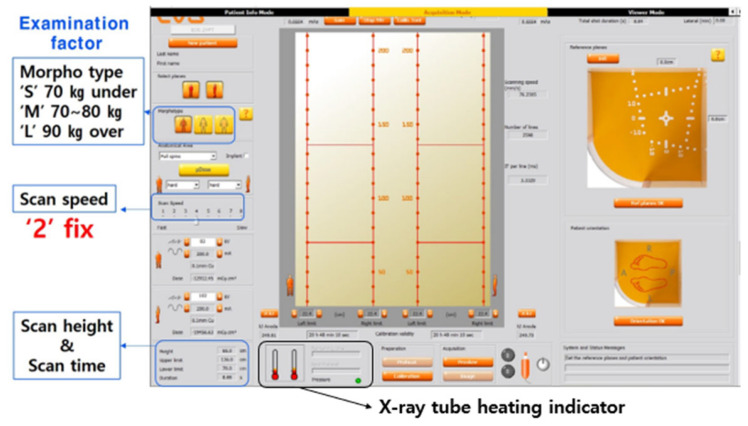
Examination factors of EOS imaging system, including morphotype, scan speed, scan height and time, and X-ray tube heating indicator.
